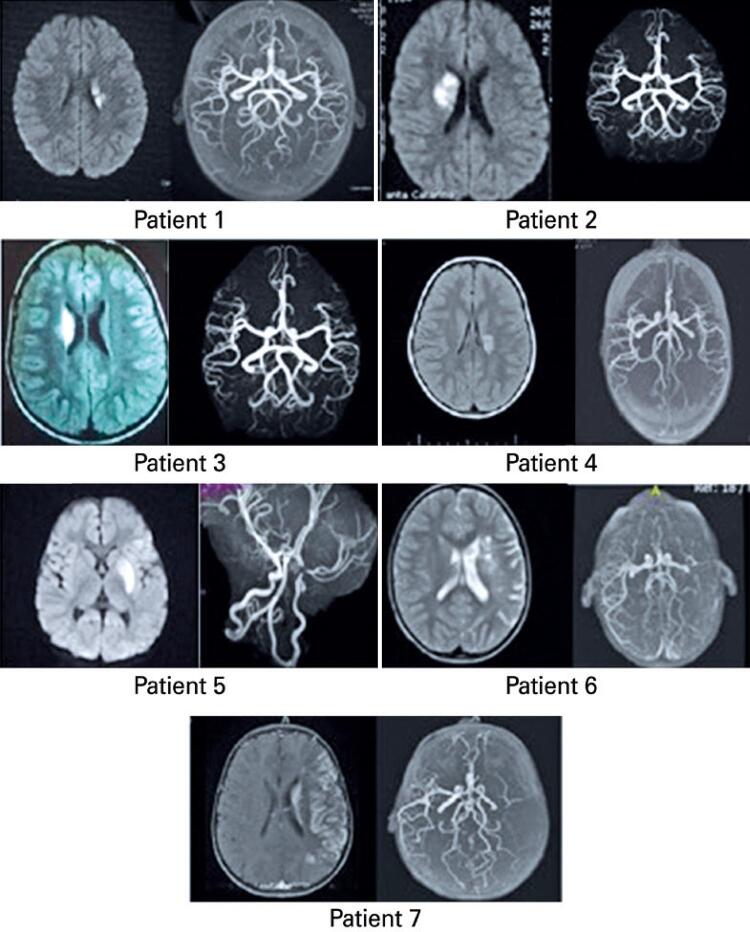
The brain magnetic resonance images from each of the seven patients with post-varicella arterial ischemic stroke
